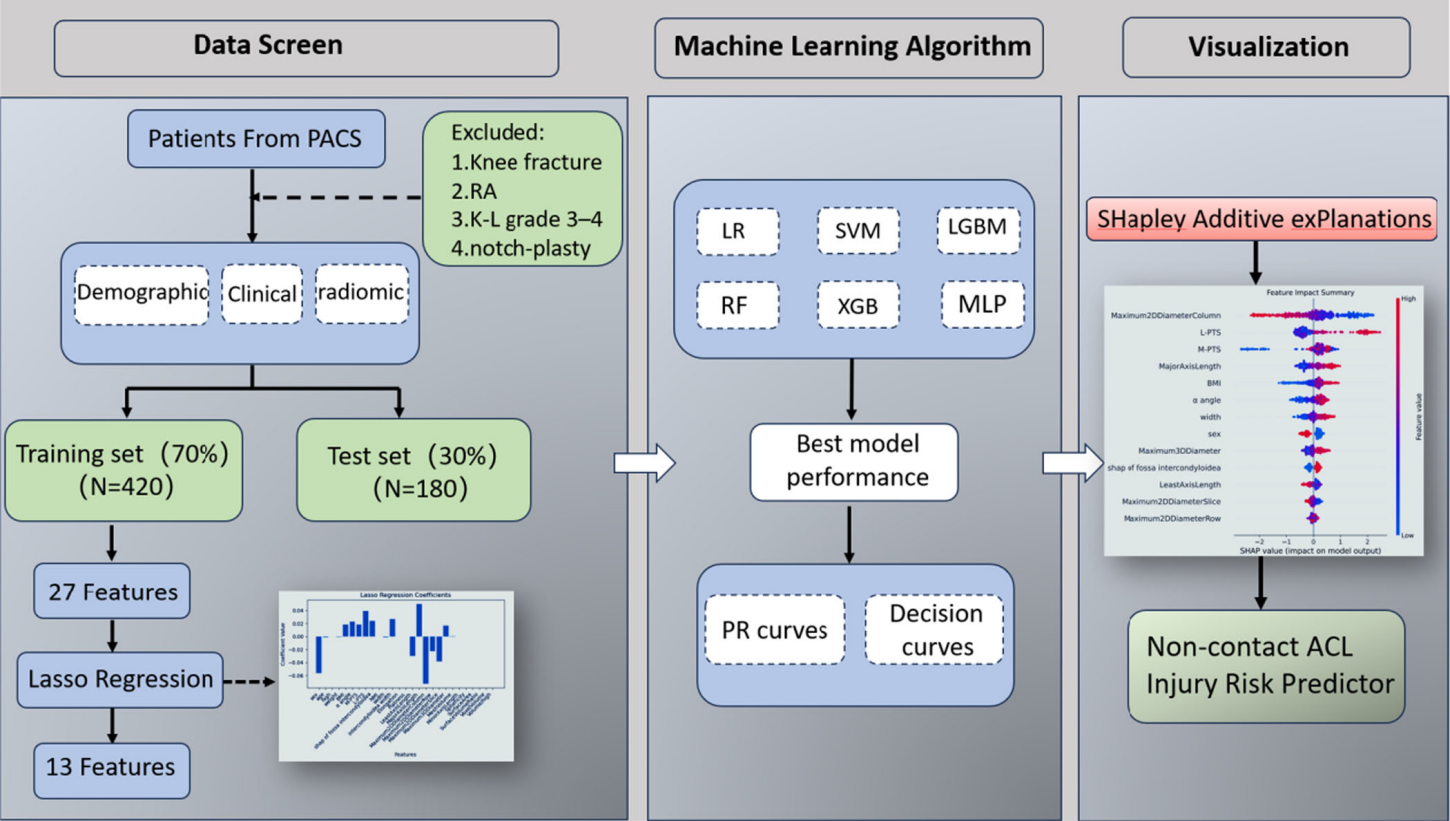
Analysis flow for the development and evaluation of the machine learning models. BMI, body mass index; LGBM, light gradient boosting machine; LR, logistic regression; MLP, multilayer perceptron; MRI, magnetic resonance imaging; PACS, Picture Archiving and Communication System; RA, rheumatoid arthritis; RF, random forest; SVM, support vector machine; XGB, extreme gradient boosting classifier.
